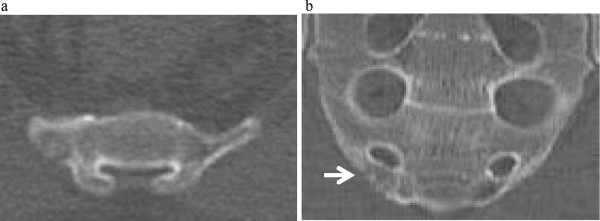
Computed tomography scan of the sacrum. The computed tomography scan shows a 9×5mm osteolytic lesion with minute mineralization in the lower end of right ala of the sacrum (arrow). (a) Axial image. (b) Coronal image.
